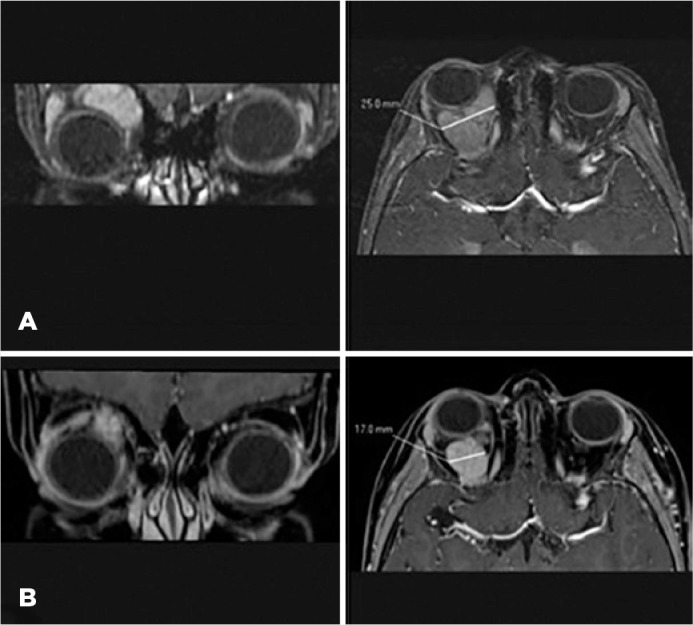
Cranial MRI, orbital section. Coronal (left) and axial (right)
